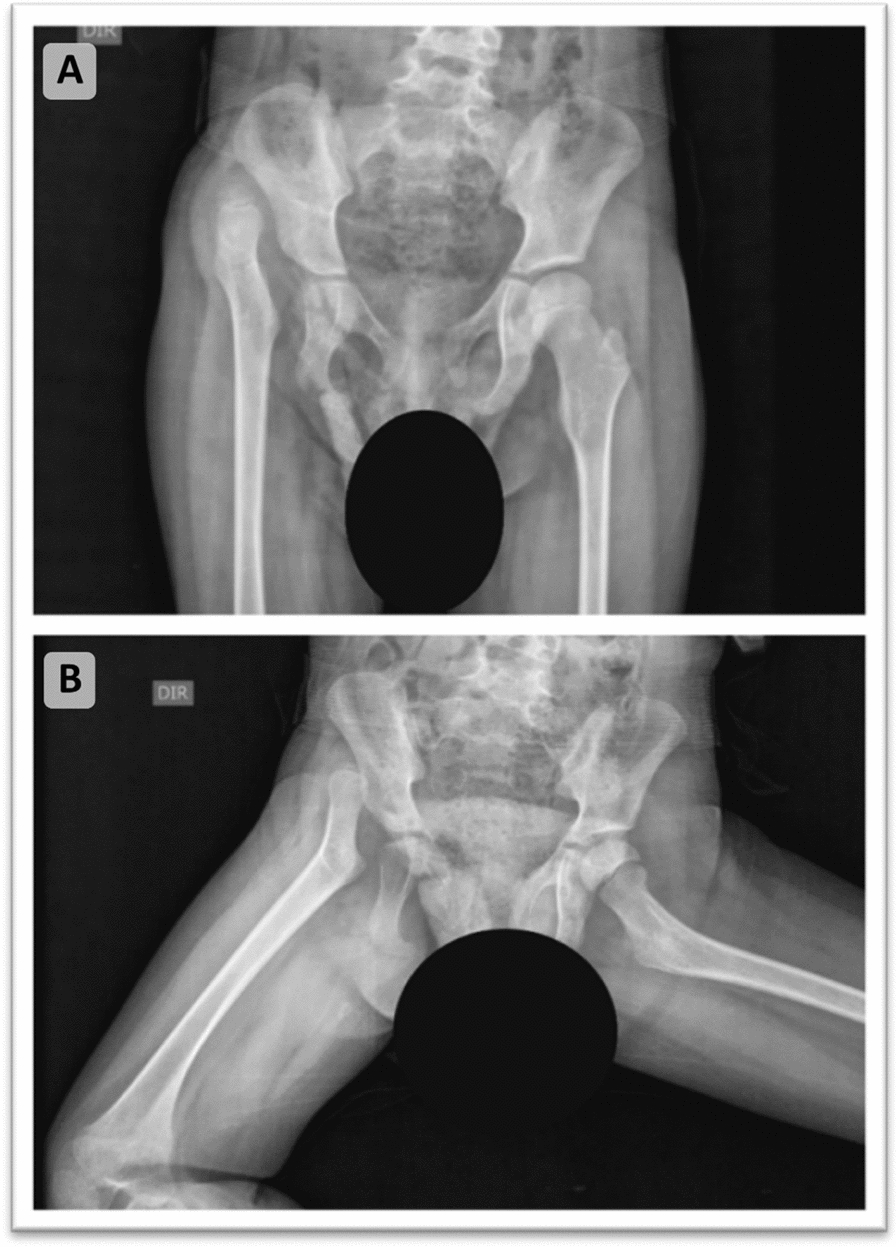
A, B* Pelvic X-ray: flattening and verticalization of the right acetabular surface, superolateral dislocation of the right femoral head, findings consistent with congenital hip dysplasia associated with right-sided dislocation in a girl with X-linked intellectual development disorder 99. *Both panels are from the same examination
